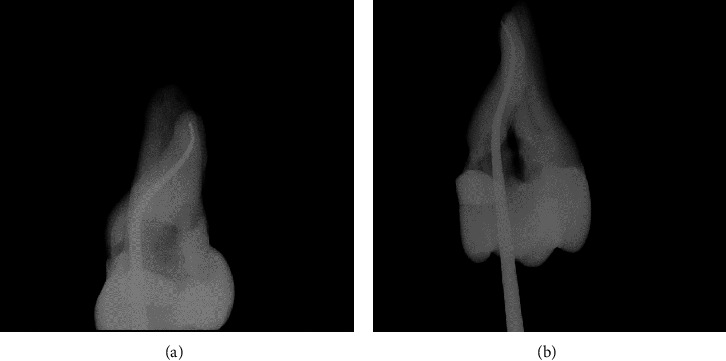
Radiographic images of an extracted maxillary second molar. (a) Gutta percha cone fitted and viewed in a buccal to lingual direction. (b) Gutta percha cone fitted in a mesial to distal view.
